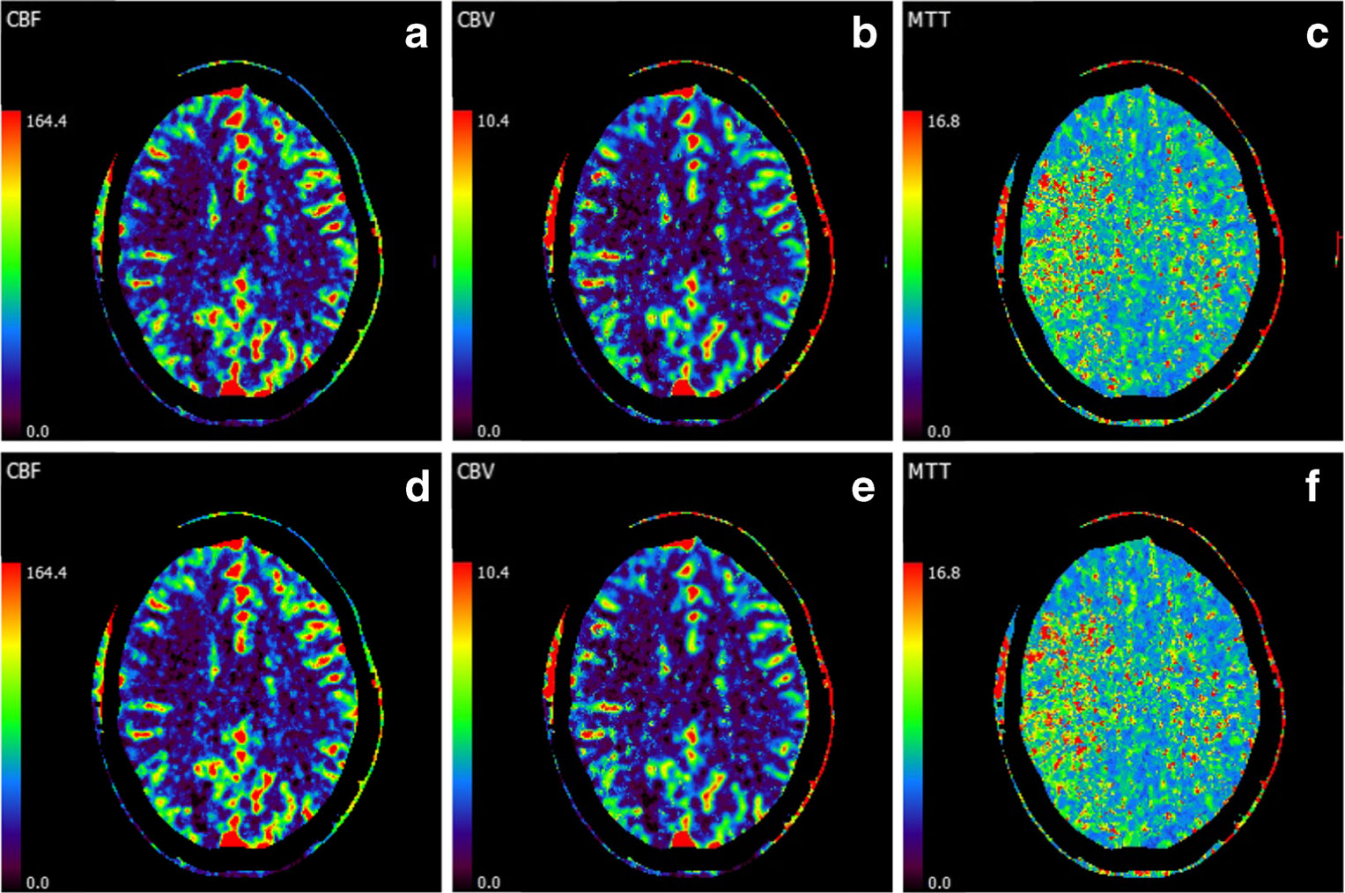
CT perfusion maps of a 41-year-old female with an infarct in the right MCA territory presented with weakness in left arm and legs and a right-sided face droop. In the upper row perfusion maps of the original CTP protocol are shown (a, b, and c). The lower row (d, e, and f) shows perfusion maps of the same patient in which the second time point was deleted. The perfusion images show an increased MTT with a small area of reduced CBV and CBF in the right MCA territory. Note that the perfusion maps are similar of the original and the perfusion maps with a 6 s gap. WL settings were not changed since the observer was shown the images as the perfusion software calculated the perfusion maps with these WL settings
